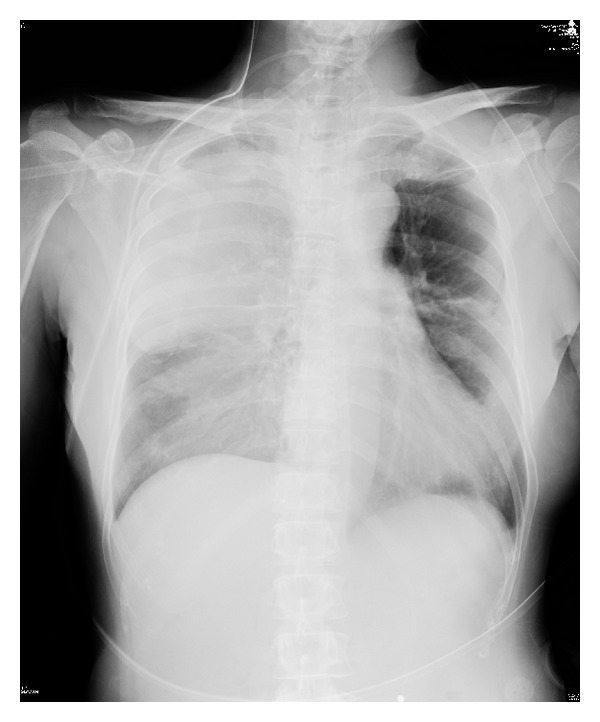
Chest X-ray (POD 4). The chest X-ray reveals a cloudy right lung and severe consolidation in the upper lobe of the lung.
